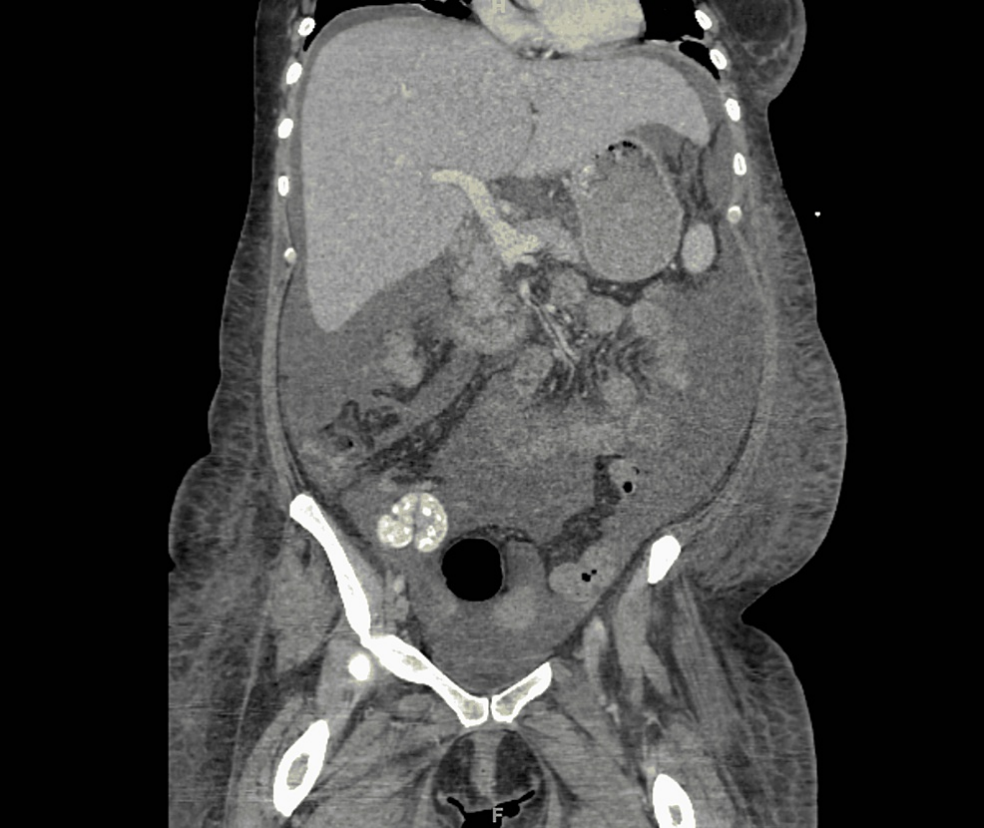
CT of the abdomen/pelvis with IV contrast (sagittal view)
